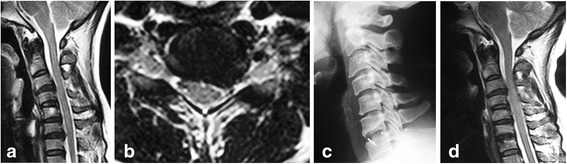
39-year-old female with C6-C7 cervical intervertebral disc herniation. a, b Preoperative T2-weighted MRI shows left nerve root compression at C6-C7 level and disc degeneration at C3-C4 level. c Radiograph after operation shows C6-C7 fusion. d MRI at 1 year after operation indicates complete decompression at C6-C7, but severe spinal cord compression can be seen at C3-C4 level
